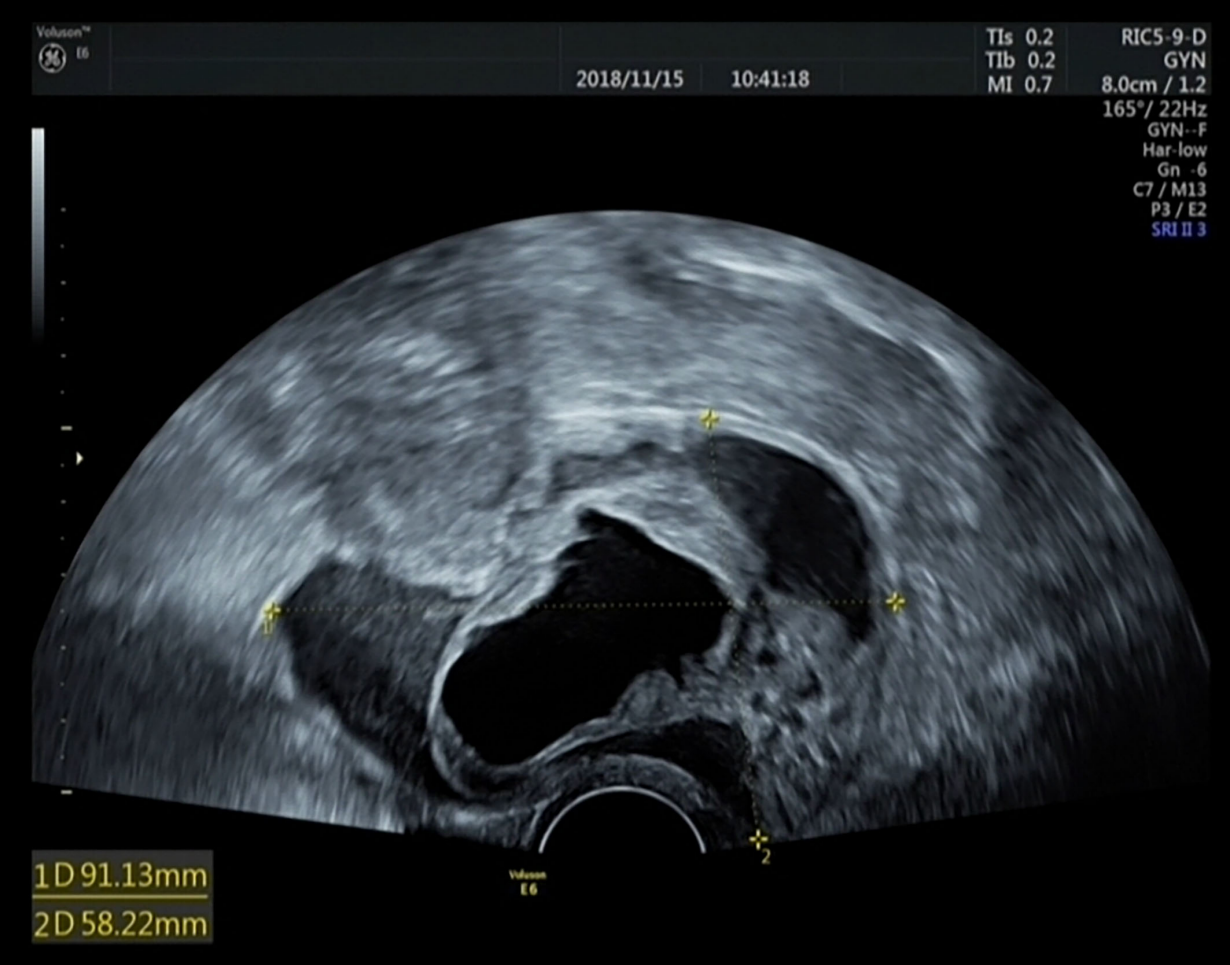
Ultrasound results of the tumor. Müllerian adenosarcoma of the uterine cervix is a cystic-solid mass measuring 9.1 × 8.1 × 5.8 cm.
